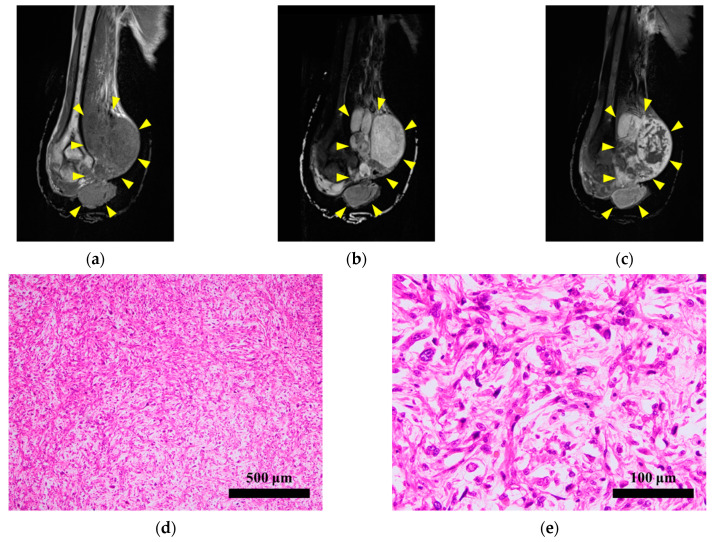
Clinical and pathological findings. Magnetic resonance imaging demonstrating a (a) low-intensity T1-weighted image and a (b) high-intensity STIR image of a soft tissue tumor around the right elbow, with a diameter of approximately 16 cm. (c) The periphery of the tumor was well enhanced by gadolinium. Yellow arrows indicate the tumor. (d,e) The tumor consisted of pleomorphic spindle cells with myxoid stroma and curvilinear vasculature.
